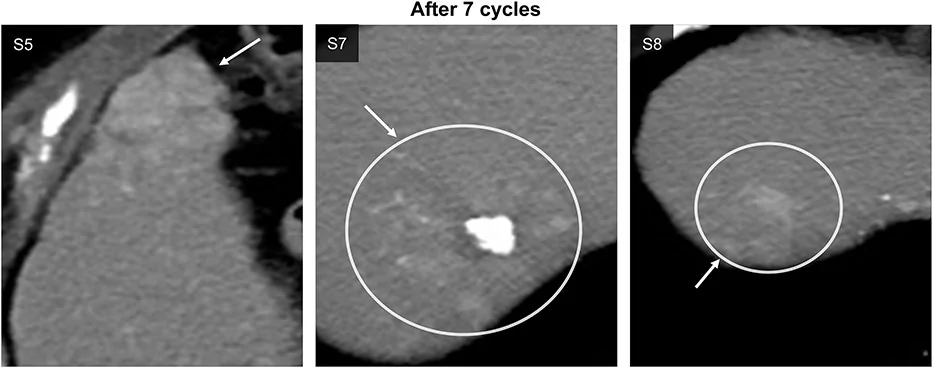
Arterial-phase CT conducted after 7 cycles of atezolizumab plus bevacizumab. Lesions in segments 5, 7, and 8 show progression with enlargement and/or expansion of enhancing areas, consistent with progressive disease by mRECIST.
